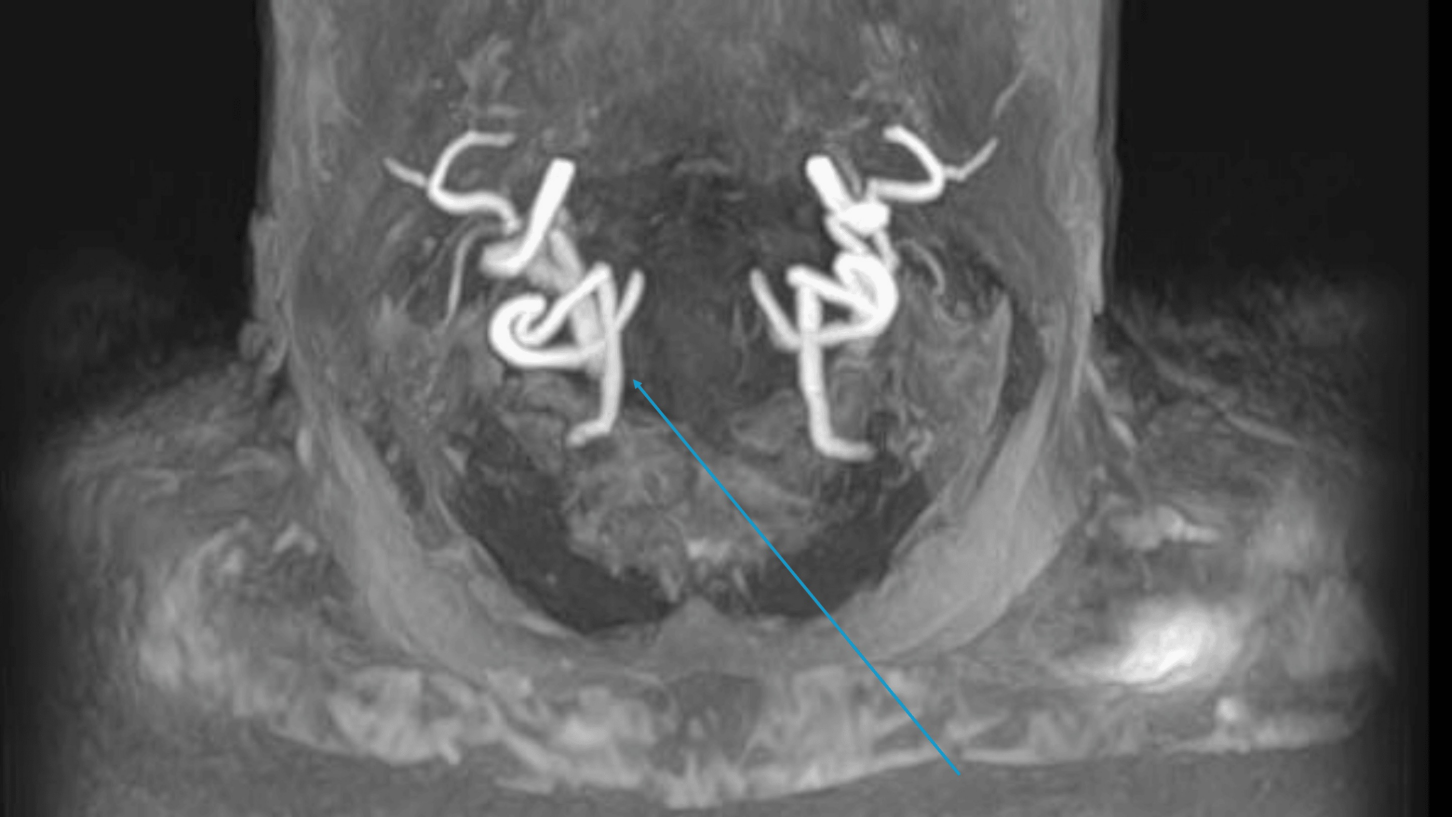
Magnetic resonance imaging of the carotids: eccentric short segment narrowing of the right internal carotid artery (blue arrow)
